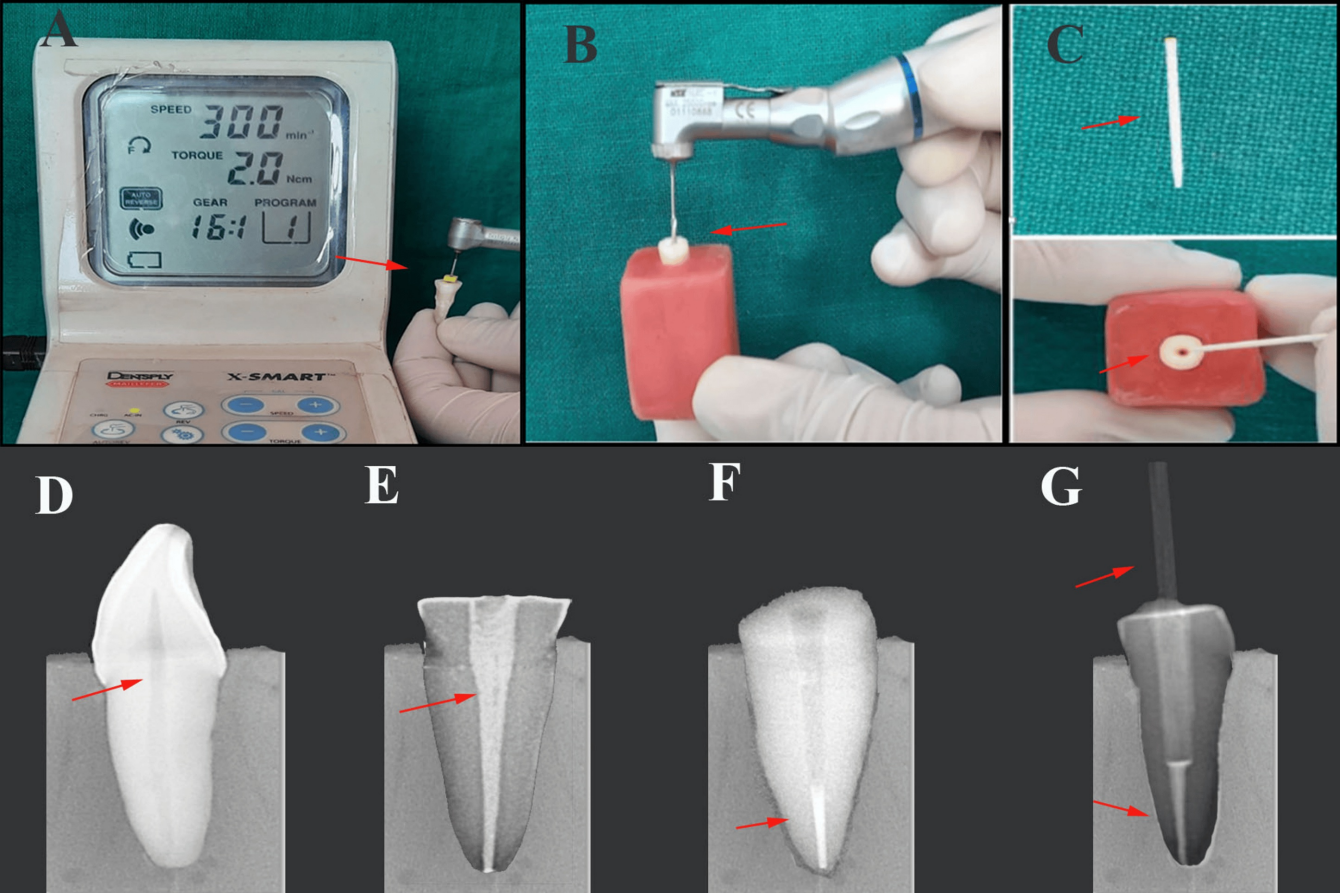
Methodology of post and core: (A) root canal preparation, (B) post-space preparation by micromotor, (C) post space for glass fiber post, (D) selected incisor tooth for post and core, (E) obturated canal with sealer, (F) post space visible on radiograph, and (G) fiber post cemented in canal Figure is derived from the study data.
